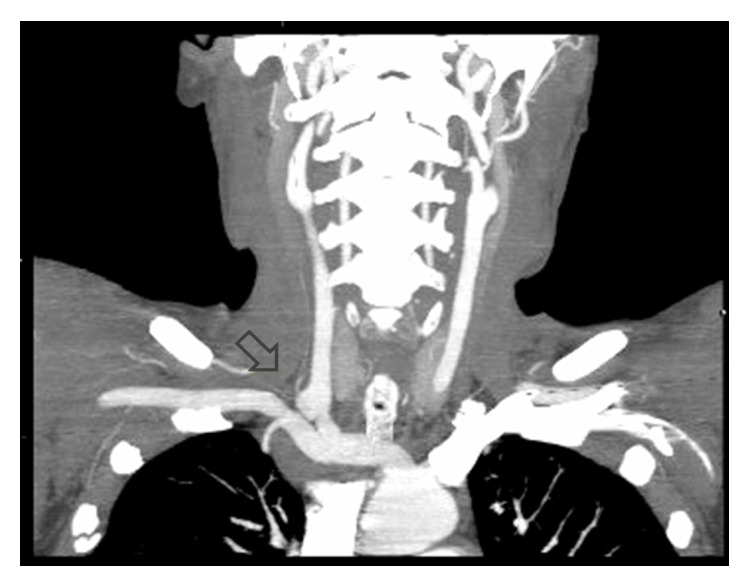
CTA shows a 10 mm pseudoaneurysm in the right common carotid artery (black arrow).
